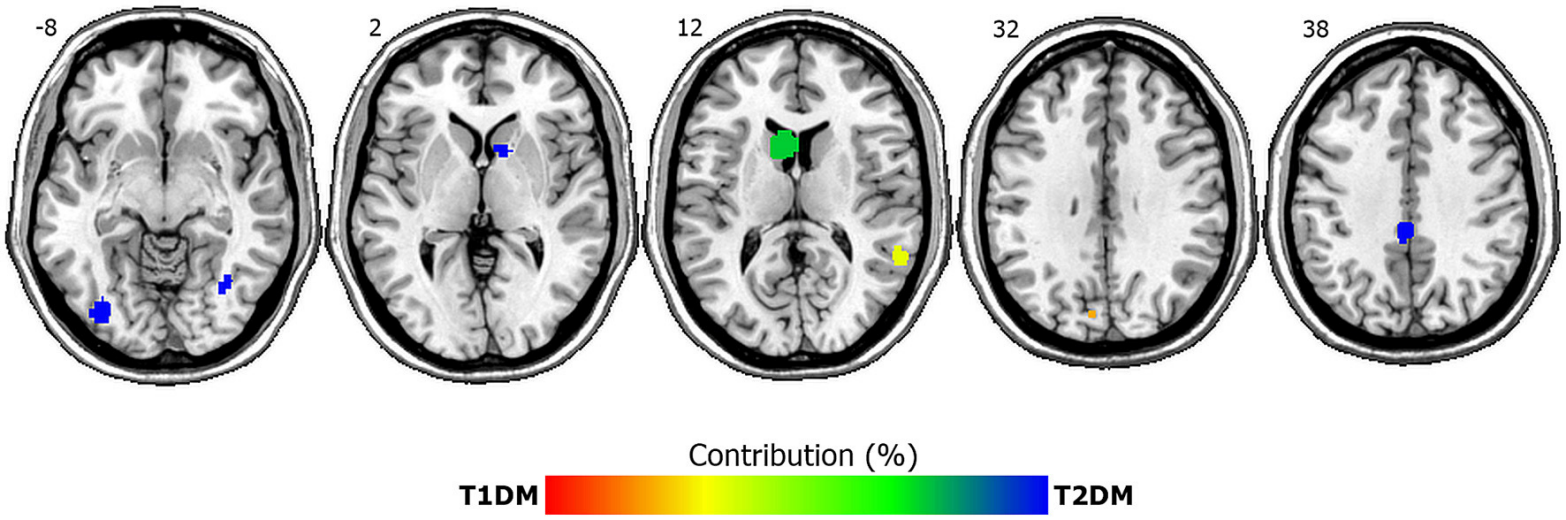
ALE clusters of lower gray matter volumes in T1DM and T2DM compared to controls. Depicted are seven significant clusters, overlaid onto axial structural MRI planes Numbers depict z-coordinates in MNI space. Blue clusters are driven by T2DM whereas both disorders contribute to green-yellow clusters. Percent contribution is signaled by the color bar, with red indicating 100% contribution by T1DM and blue indicating 100% contribution by T2DM. Left is left.
